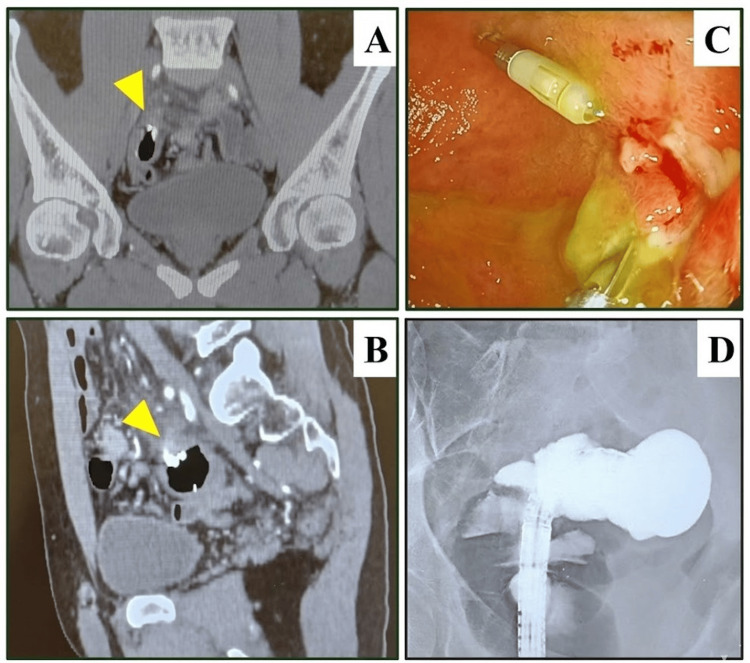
Confirmation of healing On HD 17, follow-up CT showed resolution of the pelvic abscess and deployed clips at the perforation site (A (coronal view) and B (sagittal view)). Endoscopy showed the clipped defect without an open fistula (C), and fluoroscopic contrast examination confirmed complete closure without residual leakage (D). Yellow arrowheads indicate the deployed endoscopic clips at the perforation site in A and B. HD: hospital day, CT: computed tomography
